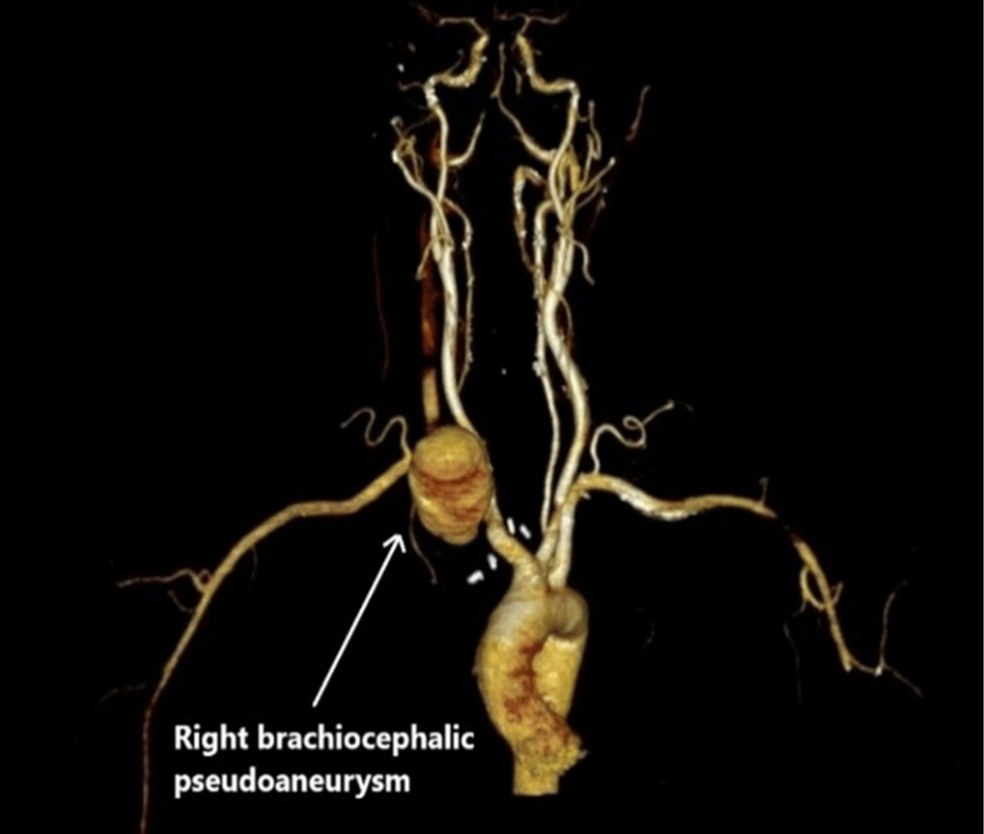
CT-angiography demonstrating right brachiocephalic pseudoaneurysm.
